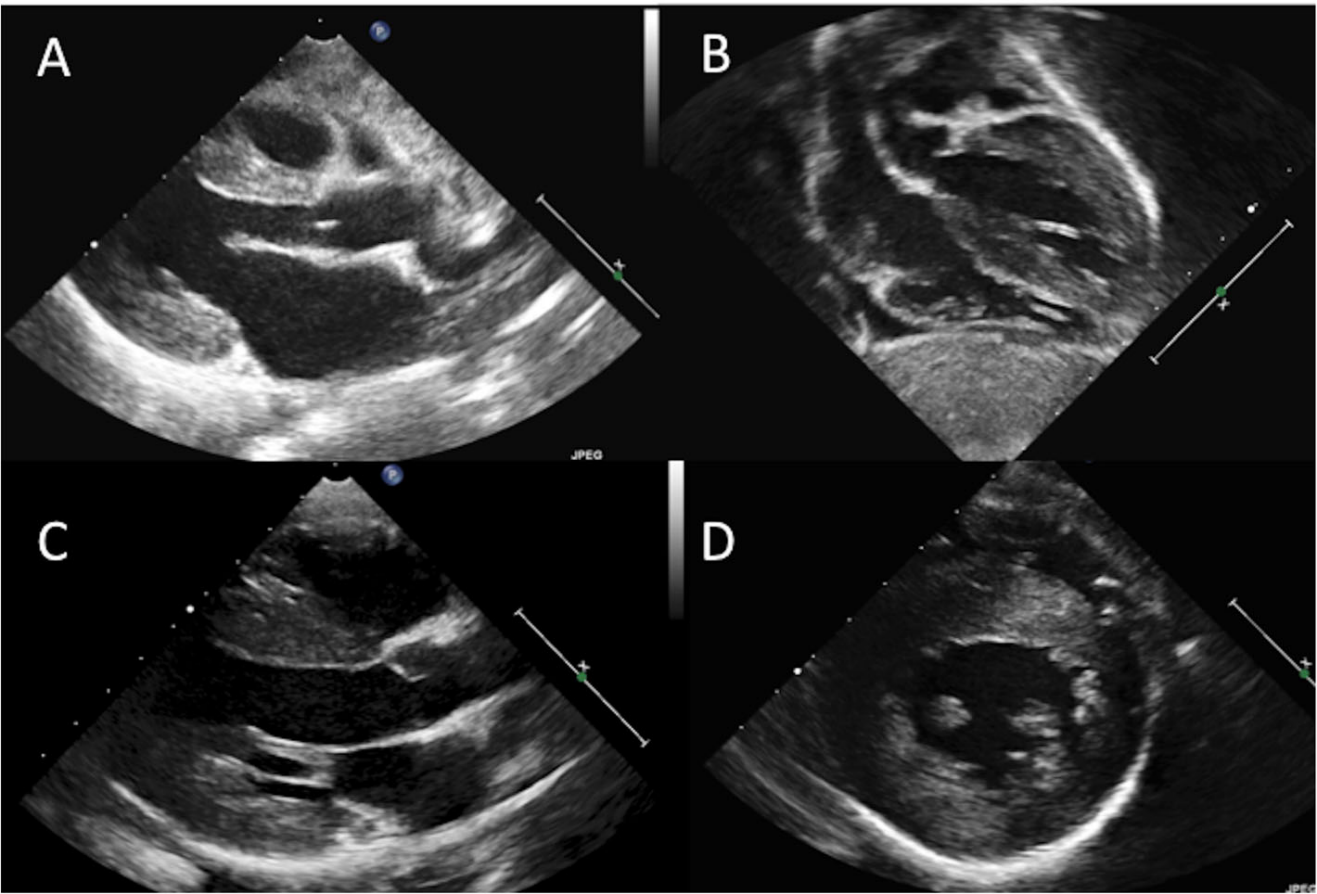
Hypertrophic cardiomyopathy with non-obstructive, concentric phenotype in a 6-month old female diagnosed with TMEM70 deficiency (Panel a: parasternal long-axis view, Panel b: sub-costal view); and in a 5-year old male with combined respiratory chain enzyme deficiencies (complex I-III-IV): Panel c (long-axis view) and d (parasternal short-axis view). All images are in diastolic phase
